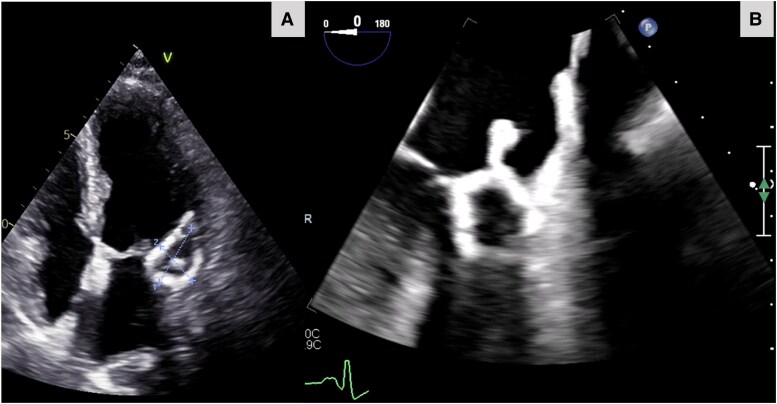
(*A*) Transthoracic echocardiography (apical four-chamber view) demonstrating the posterior mitral annular mass. (*B*) Transoesophageal echocardiography confirming the lesion and revealing a highly mobile atrial-side component.
